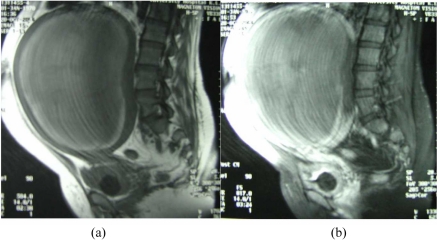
Pre-gadolinium-DTPA sagittal T1-weighted image of MRI pelvis (a) and post-gadolinium-DTPA image (b) demonstrating a large homogeneous low signal intensity lesion in the anterior uterine wall (arrow) in keeping with fibroid, which does not enhance following contrast administration. Motion artifacts are noted on post-gadolinium sequences showing in Figure 3(b).
